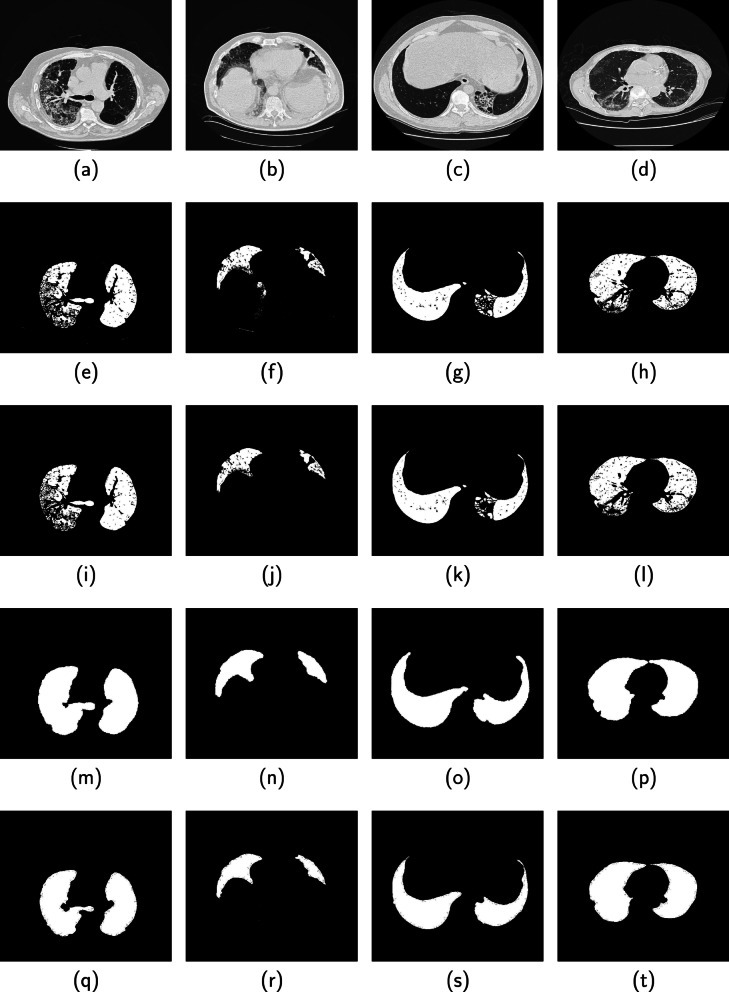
Another performance of the proposed method on four CT images from the ILD database are displayed in columns 1, 2, 3 and 4. (Row 1) Original image, (Row 2) Binarized image, (Row 3) Candidate lung region, (Row 4) Segmented lung region (Row 5) Groundtruth
